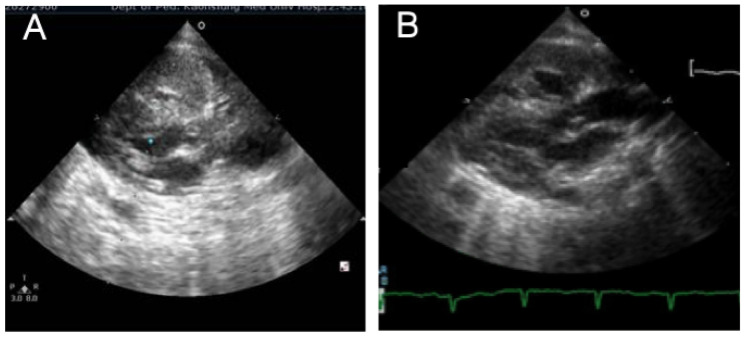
Long-axis echocardiographic image in a 1-day-old female newborn revealed a hypertrophic right ventricle without patent ductus arteriosus noted (A), which improved 5 days later (B).
